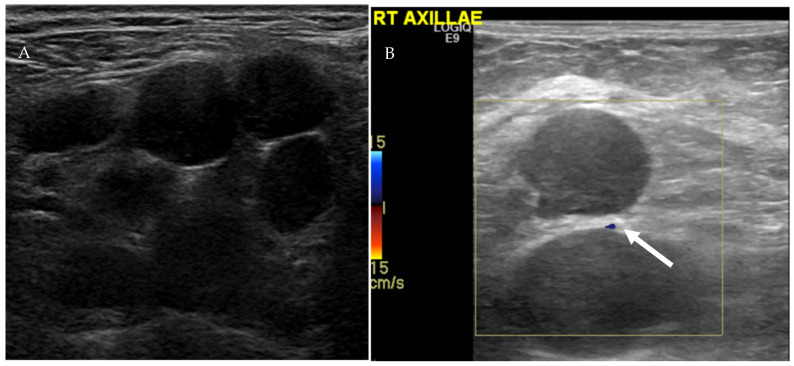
Ultrasound of the right axilla (A) demonstrates multiple, round, and hypoechoic metastatic lymph nodes with S/L axis ratio of approximately 1 and lack of the echogenic fatty hilum. Color Doppler ultrasound of the axillary lymph (B) nodes shows peripheral vascularity (arrow).
